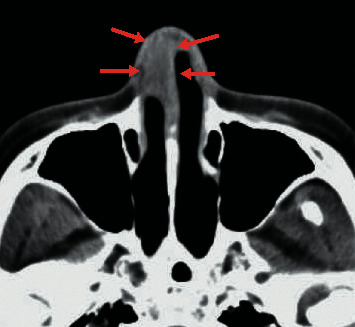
Computed tomography scan revealed a mass lesion localized in the anterior part of the right nasal cavity (arrow).
